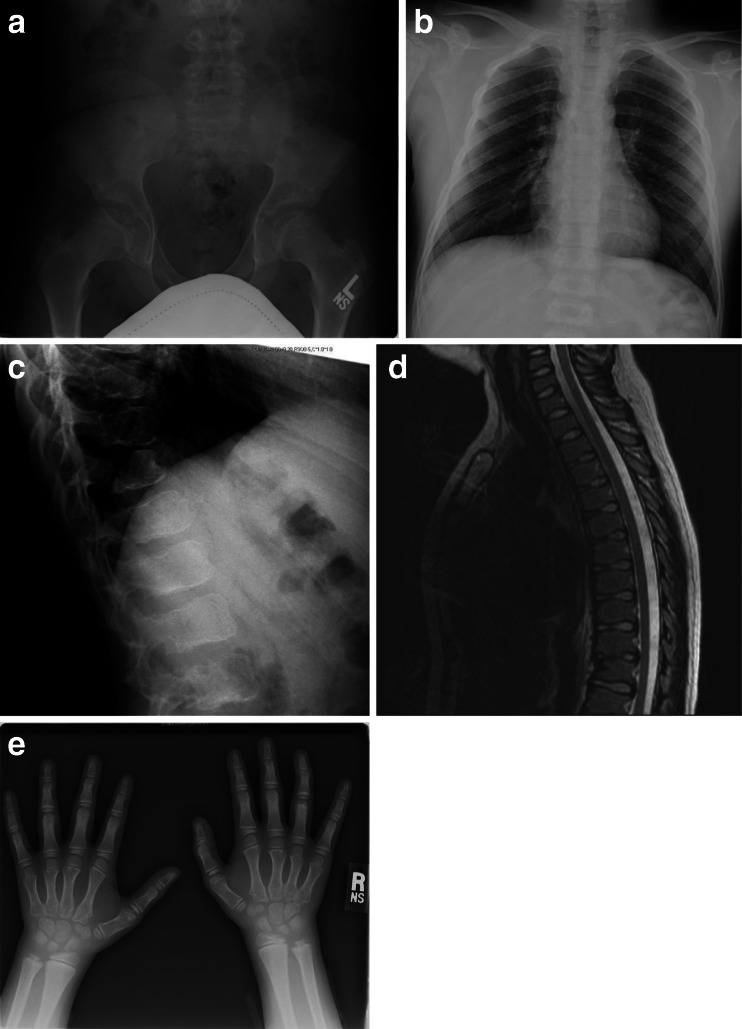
Radiographs and MR image of patient 8, male, MPS VI. a AP pelvis and hips, age 12 years: hypoplastic CFE. b AP chest, age 12 years: normal. c Lateral spine, age 12 years: superior notched T12 vertebral body. d MRI lateral spine, age 12 years: anterior/superior vertebral body irregularity/notching with intact region of cartilaginous anlage. e AP hands and wrists, age 10 years: normal
